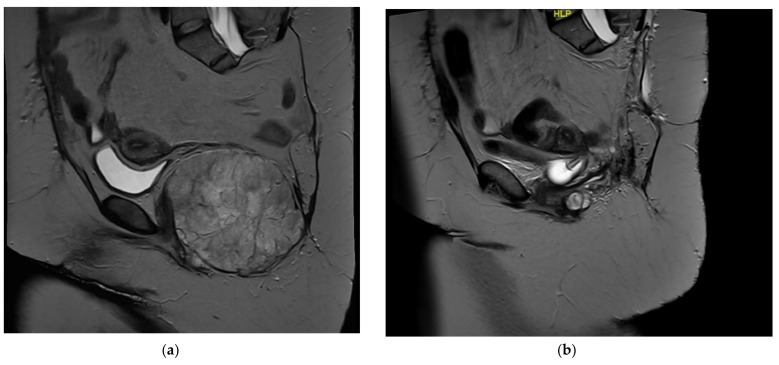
(a) Sagittal T2 MRI demonstrating a recurrent chordoma in the pelvic floor with evidence of prior sacrectomy. (b) Postsurgical sagittal T2 MRI demonstrating postsurgical changes after en bloc resection of chordoma requiring removal of the distal colon, rectum, and anus.
